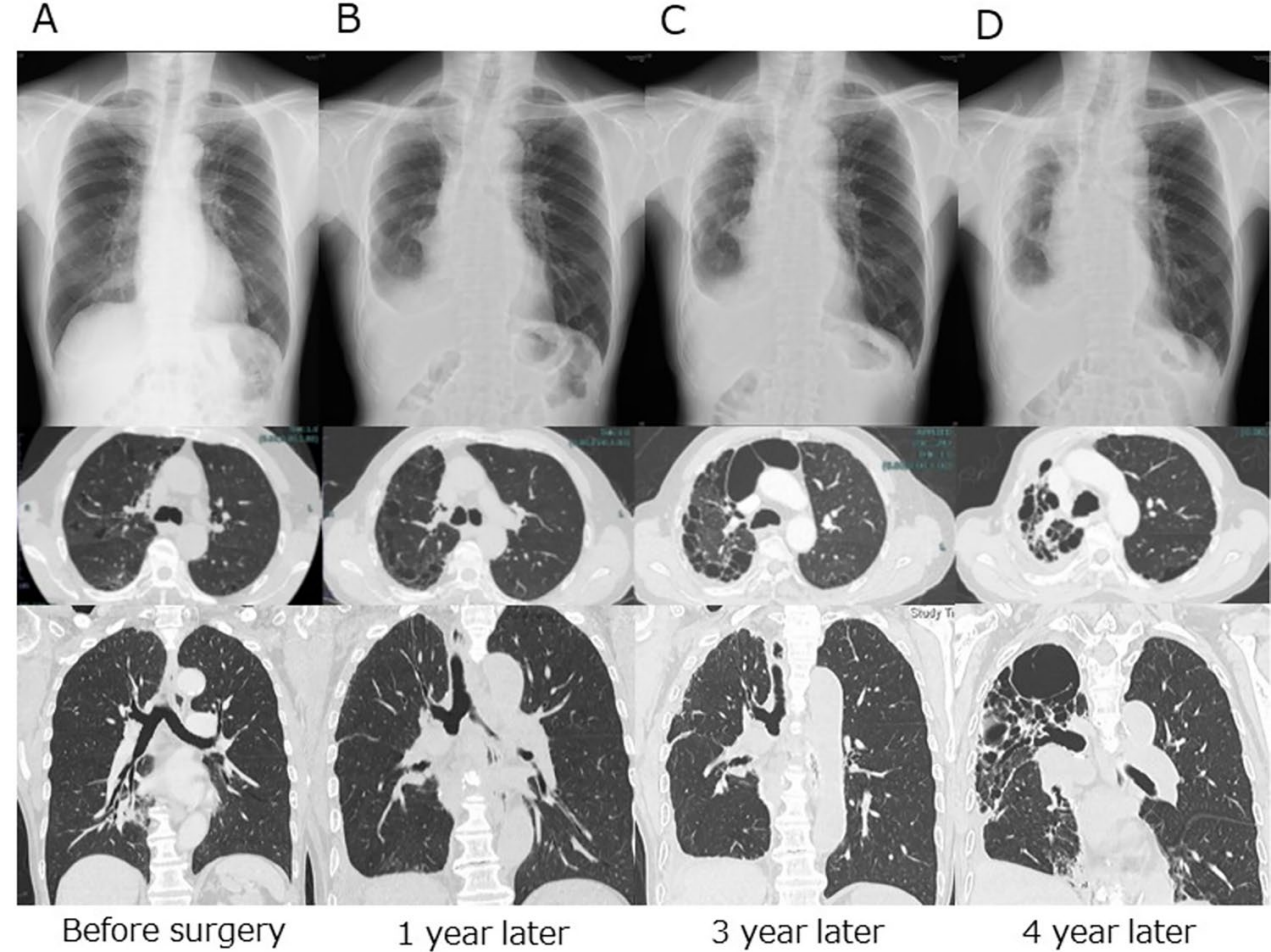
Radiological course of a 74-year-old male patient who underwent right lower lobectomy for stage IA1 adenocarcinoma via VATS. A Before surgery, there is no mediastinal shift. B Postoperative chest X-ray and computed tomography one year after surgery showing right pleural effusion. C Three years after surgery, a subpleural parenchymal lesion in the right upper lobe showed slight fibrosis with pleural spicula or indentation and a mediastinal shift. D Four years after surgery, the cystic lesion in the right upper lung field is obviously deteriorated with unilateral thoracic deformity
